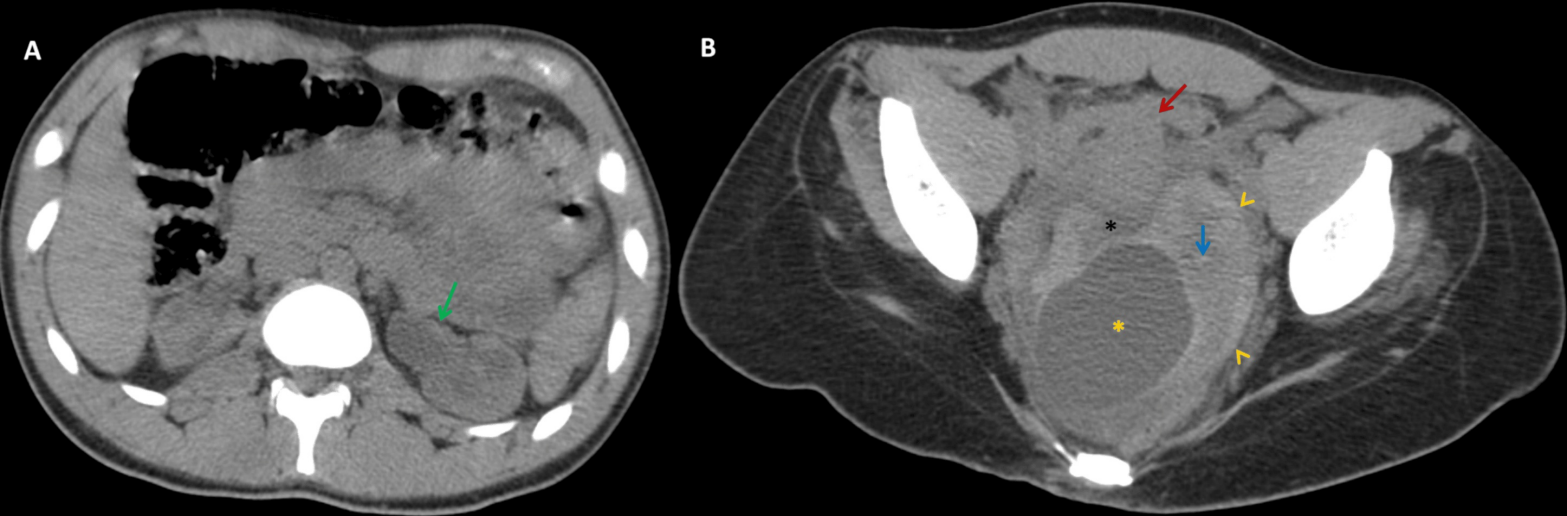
Axial non-contrast CT imaging of a pelvic mass and left urinary tract dilation A. Axial CT slice at the level of the renal hilum demonstrating left pyelocaliectasis (green arrow), indicative of upper urinary tract dilation. B. Axial CT slice at the pelvic level revealing the previously identified ultrasound mass, appearing as a reniform structure mimicking an ectopic kidney. The lesion exhibits a spontaneously hyperdense periphery (yellow arrowheads) and a hypodense central region (blue arrow), with an associated large cystic component (yellow asterisk). The uterus is visualized anterior to the mass (red arrow), along with free pelvic fluid (black asterisk).
